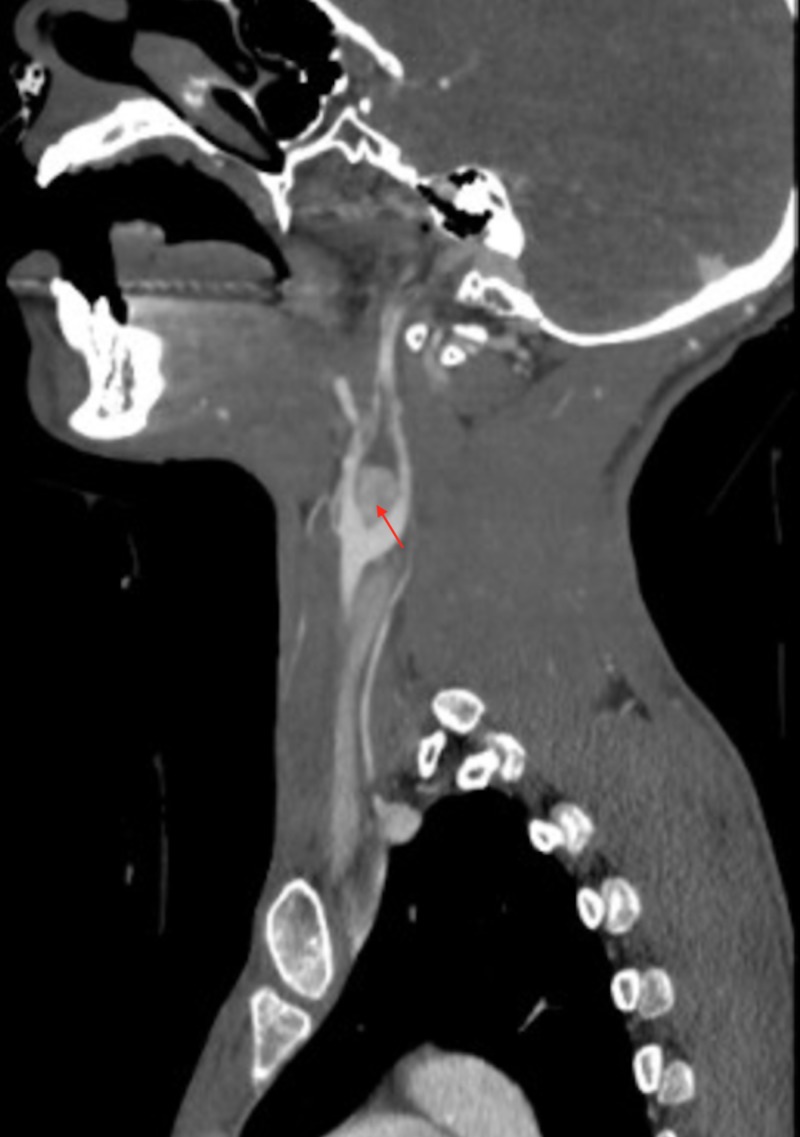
Sagittal view of computed tomography angiogram of the neck demonstrating an enhancing mass, approximately 13 mm in diameter, splaying the left internal and external carotid artery, consistent with paraganglioma
